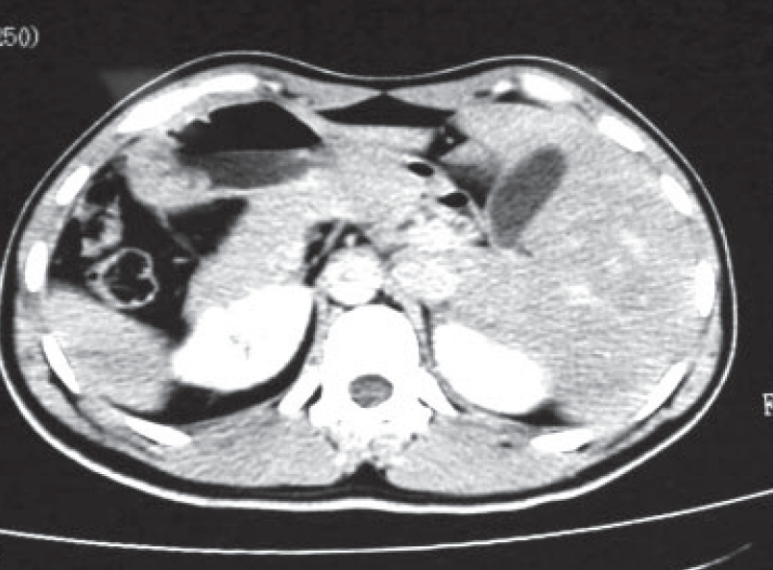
CT scan of situs inversus
